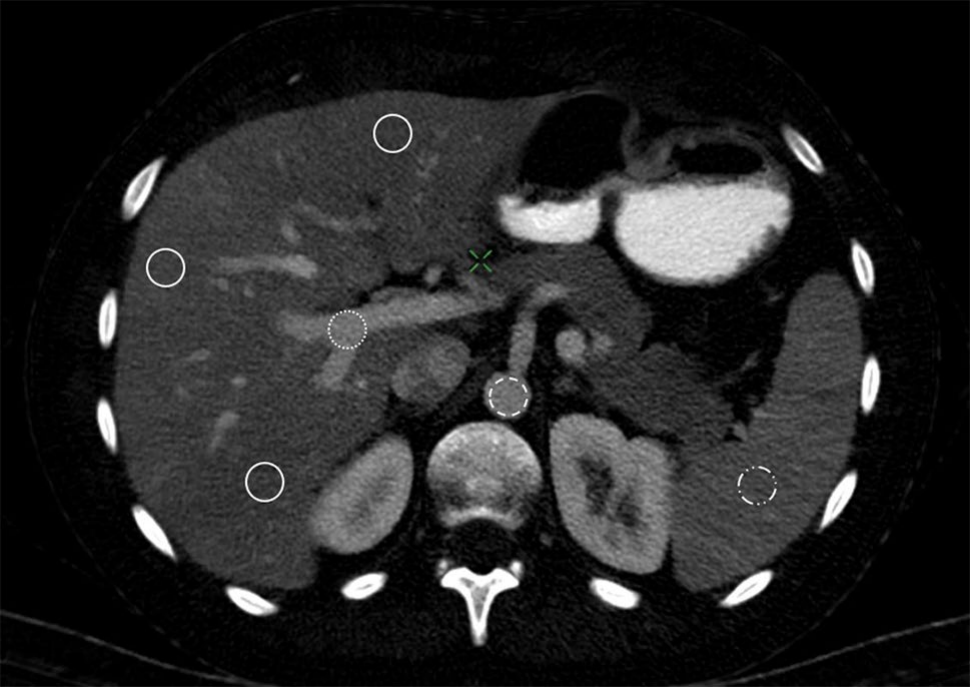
Placement of the ROIs in a transversal plane dual-energy CT quantitative iodine image of a 55-year-old male individual. The portal venous phase DECT iodine image corresponds to the coeliac axis and shows a total of six ROIs. Three ROIs are placed in the hepatic tissue (continuous line), one in segment 3 in the left hepatic lobe and two in the right hepatic lobe in segments 4b and 7. One ROI (dotted line) is in the portal vein, and one ROI (dashed line) is in the aorta. Another ROI is placed in the spleen tissue (dotted and dashed line)
